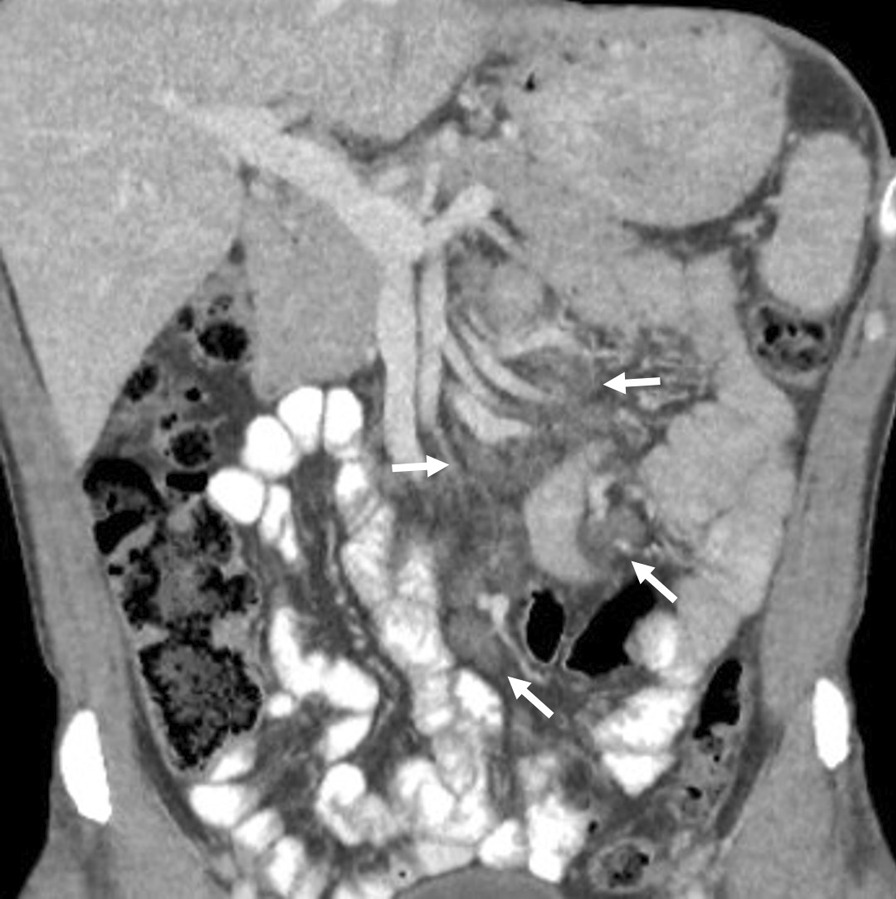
Coronal CT showing enlarged lymph nodes in the small bowel mesentery (white arrows). All of them have very low attenuation suggesting fat content which is common in Whipple’s disease, Patient 3
